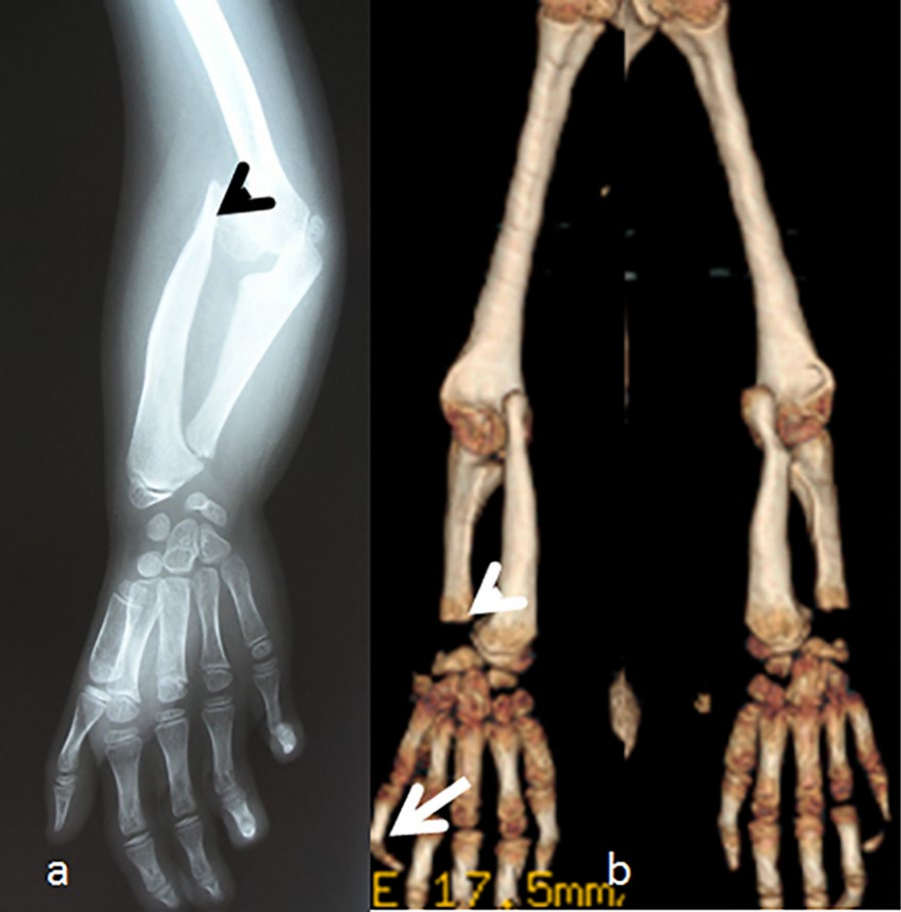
Radioulnar dislocation and severe retardation of the fine motor skills. Anteroposterior radiograph of the upper limb in a 7-year-old child. Note the marked mesomelia and the superior and inferior radioulnar dislocations (arrow head) with Madelung’s-like deformity associated with camptodactyly of the fourth and fifth fingers, respectively (a). Three-dimensional (3D) reconstruction computed tomography (CT) scan of the upper limbs in a 5-year-old girl with autosomal recessive Robinow syndrome showed bilateral radioulnar superior and inferior dislocation (Madelung’s deformity—arrow head) with marked dysplastic process over the epi metaphyseal components, small hands with bilateral camptodactyly of the fourth and fifth fingers (arrow). All patients with autosomal recessive Robinow syndrome presented with marked limitations in pronation and supinations because of mesomelic dysplasia secondary to Madelung’s deformity (b).
